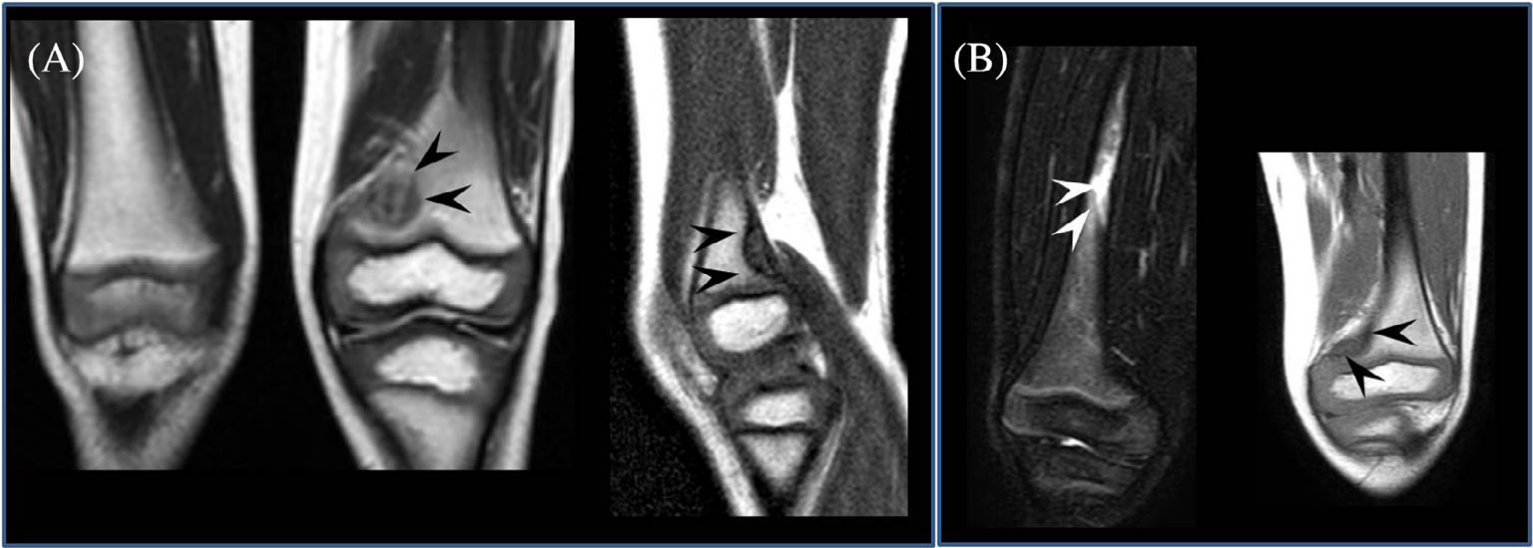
MRI of the femurs before the start of therapy (A) hypointense lesion in T1‐weighted sequences at the meta‐diaphyseal region of the left femur (12 × 4 × 13 mm) also with evidence of sclerotic border (black arrows). (B) 3 months after the first MRI, time 0 at the start of ERT: appearance of confluent infarction lesions hyperintense in T2‐weighted sequences (total max diameter 3 cm) in the central area of the diaphysis of right femur (white arrows). Stable hypointense lesion in T1‐weighted sequences in the left femur (black arrows).
